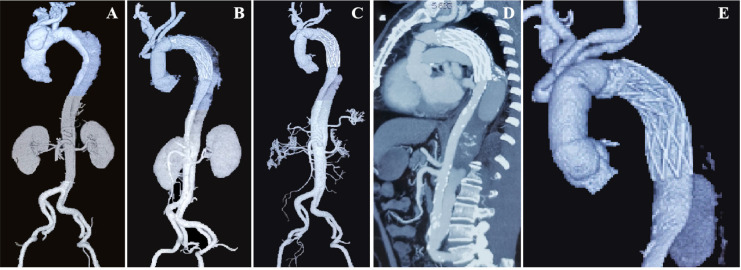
Computed tomography angiography images. (A) Before operation, (B) after
